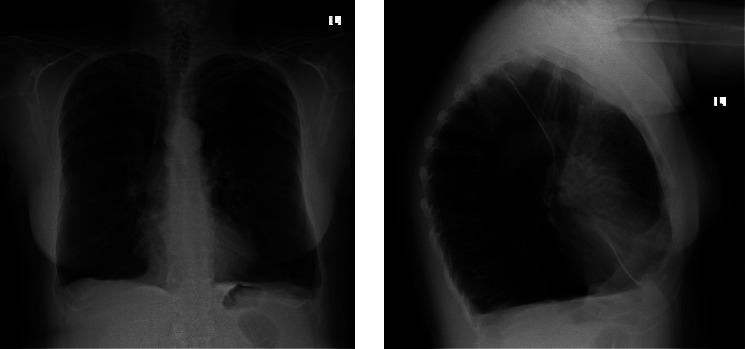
Chest X-rays of posterioanterior and lateral view showing bilateral lung hyperinflation, diaphragm flattening, and hyperlucency.
